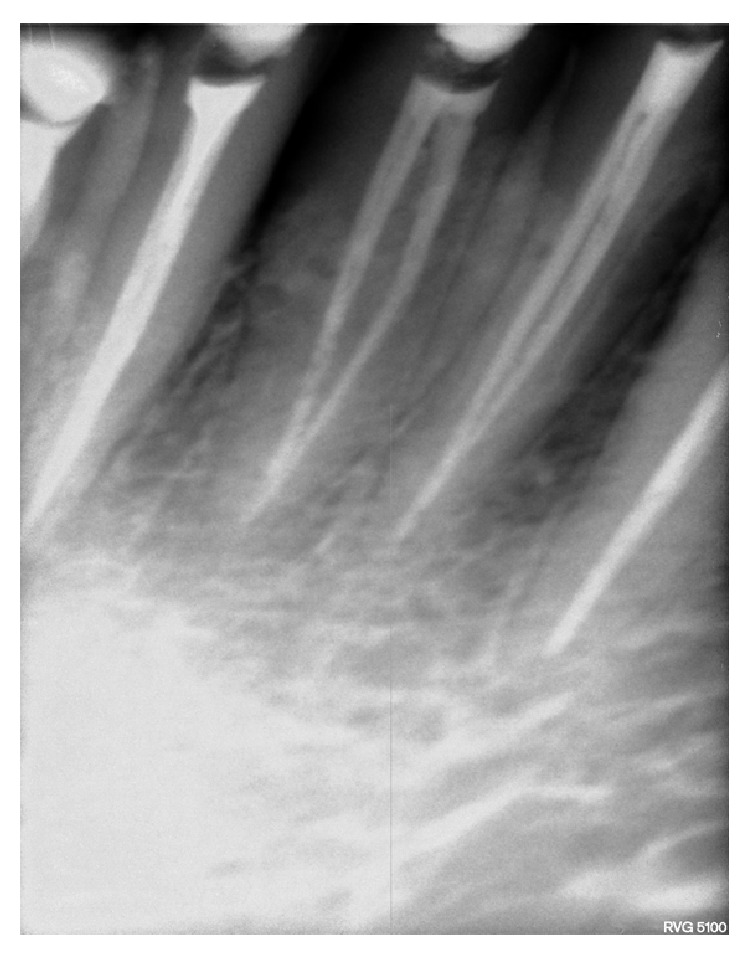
Radiograph showing obturation in two separate canals in 41 and 42.
